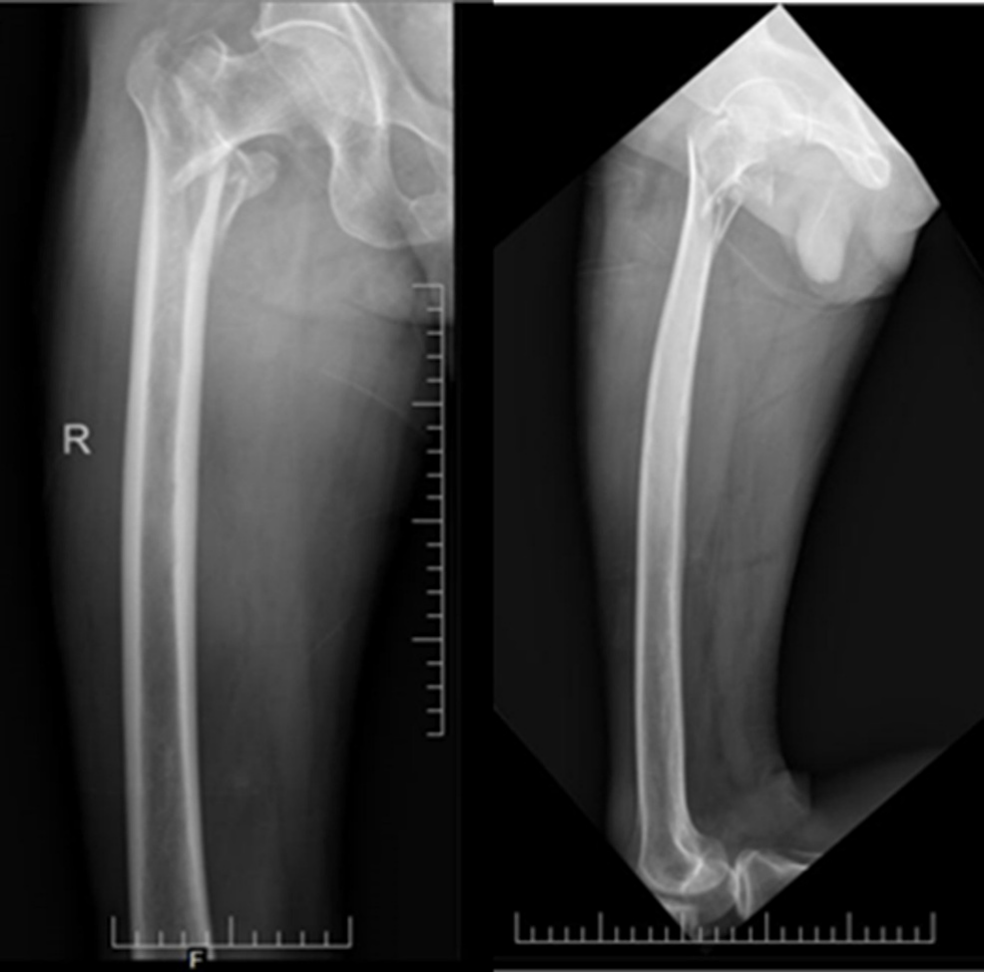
Right hip radiograph - AP (L) and lateral (R) views
